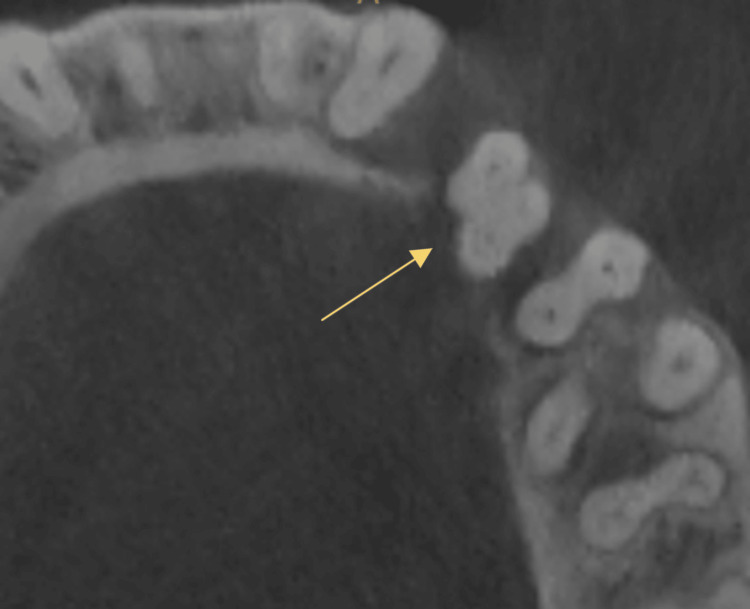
CBCT image showing one mesial and two distal canals of case 1
